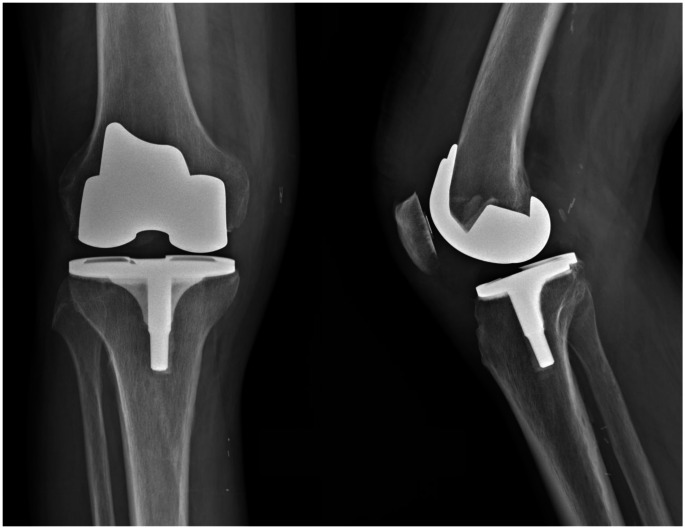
X-rays of a 79-year-old male individual with Parkinson’s disease, taken one year after surgery. The total knee arthroplasty (TKA) was performed using a functional alignment approach. At the follow-up, the patient demonstrated excellent clinical and functional outcomes, regaining full independence in daily activities. No signs of radiographic loosening of the implant can be found
